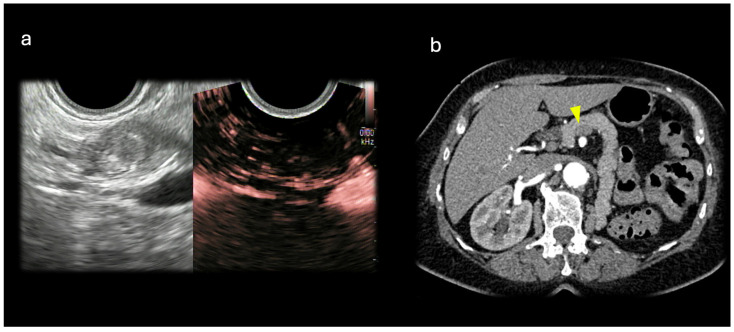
Representative post-ablation imaging assessment of the same treated lesion, demonstrating lack of residual vascular enhancement on EUS with DFI assessment (a) and on contrast-enhanced CT (b); yellow arrowhead.
